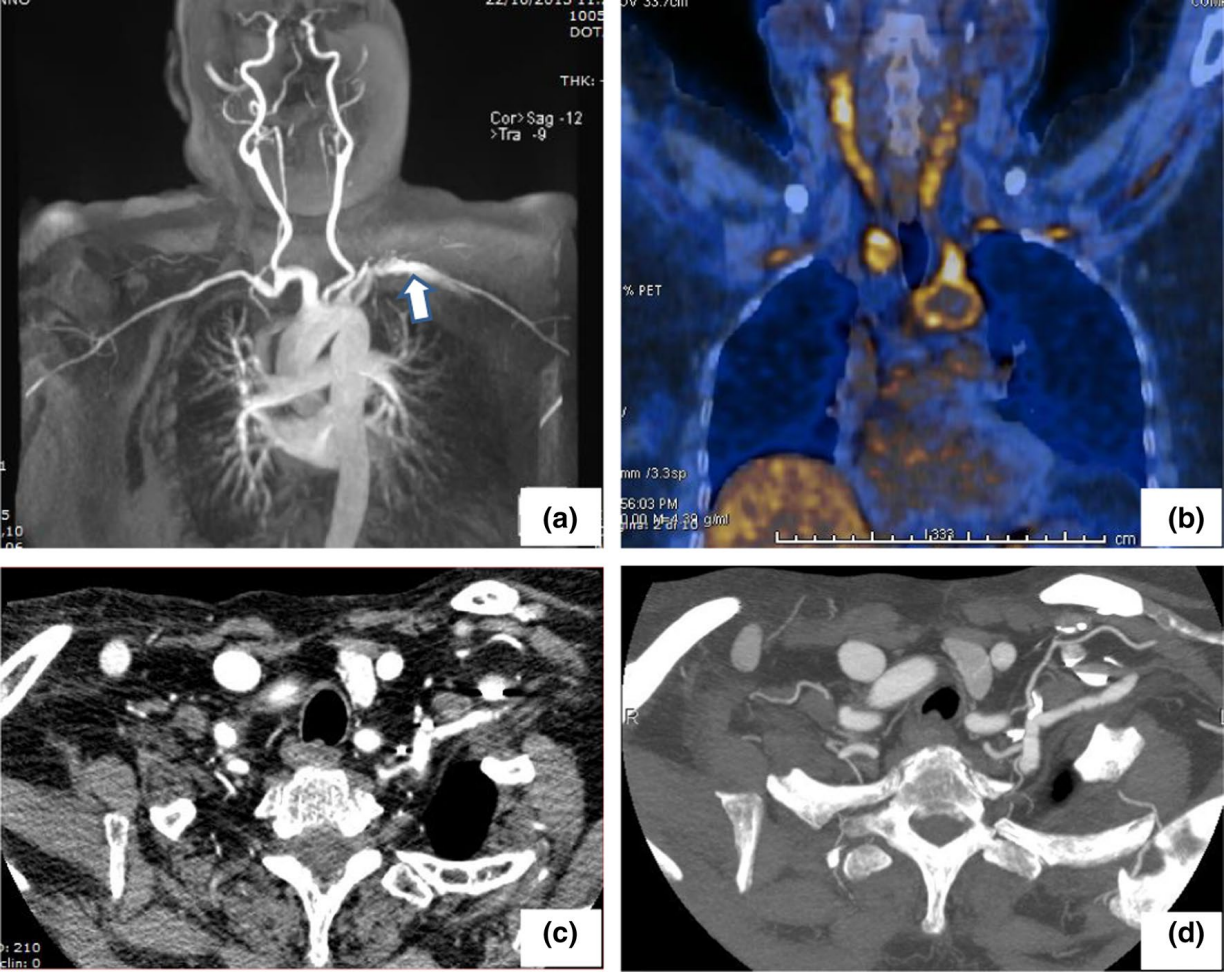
a Angio-MR with intravenous paramagnetic contrast medium shows a moderate ectasia and an irregular contour of the proximal tract of left subclavian artery (arrow). b By ^18^FDG-PET/CT an increased uptake of the radiopharmaceutical can be seen at the level of the neck vessels and thoracic aorta. c Following therapy, CT with intravenous iodinated contrast medium reveals a reduced irregularity of the left subclavian wall and a more homogeneous gauge. d The same CT with maximum intensity projection (MIP) algorithm
